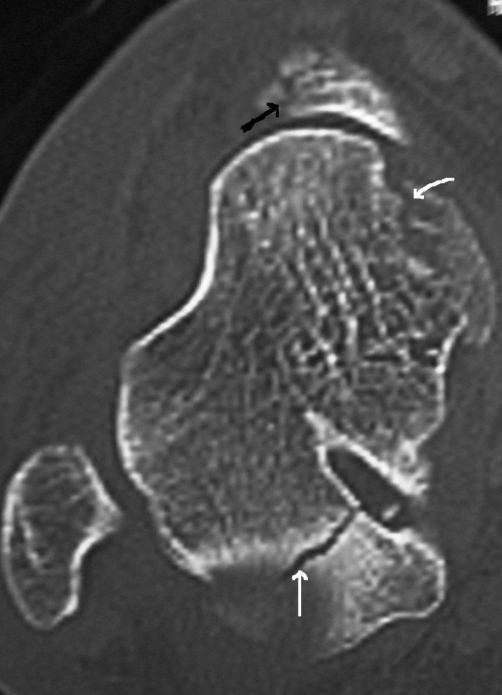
CT scan of the right foot showing two osteochondral fractures of the talus (white arrows) and an undisplaced navicular fracture (black arrow).
